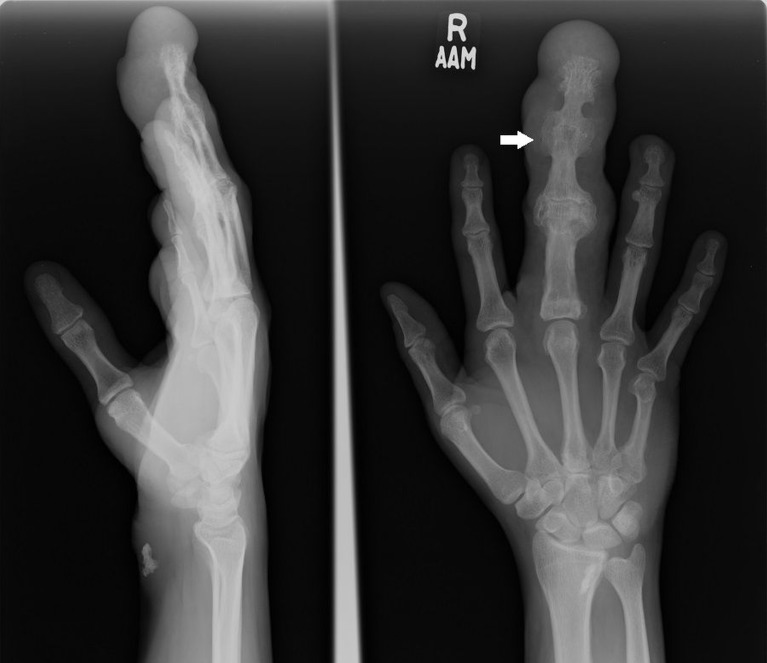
Right hand radiograph shows the lengthening of the middle finger (arrow) with a splaying and broadening of the middle phalanx due to secondary degenerative changes and diffuse soft tissue swelling.
